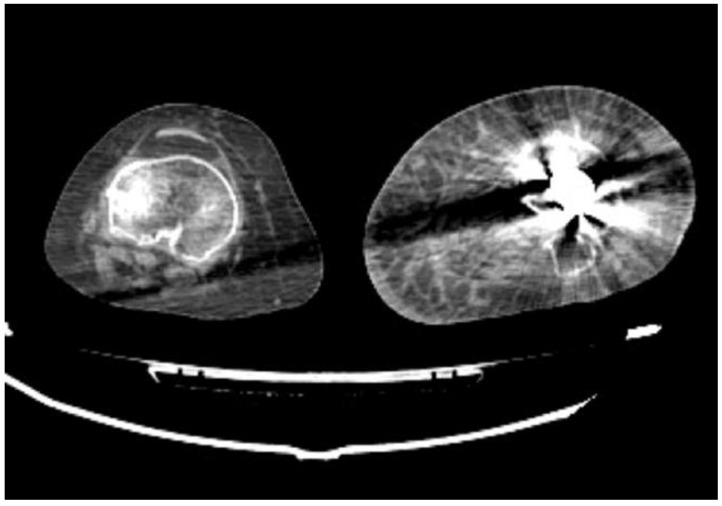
CTA, arterial phase, axial planes. In this patient, artifacts from metal arthroplasty of the left lower limb made the examination nondiagnostic. An iterative filter must be applied in order to reduce these artifacts.
